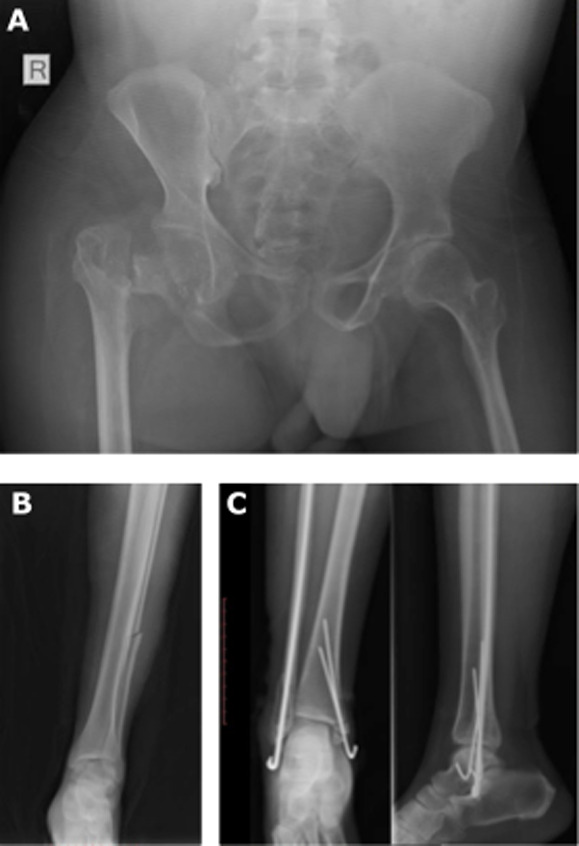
sample patients with inadequate treatment; A) unavailable implants: basicervical femoral neck fracture status-post excisional arthroplasty instead of open reduction internal fixation/total hip arthroplasty; B) incomplete injury evaluation: distal femur/midshaft fibular fracture without appropriate evaluation of ankle and syndesmosis; C) intraoperative fluoroscopy unavailable: postoperative radiographs of bimalleolar fracture demonstrate medial malleolar fragment not captured by k-wire
